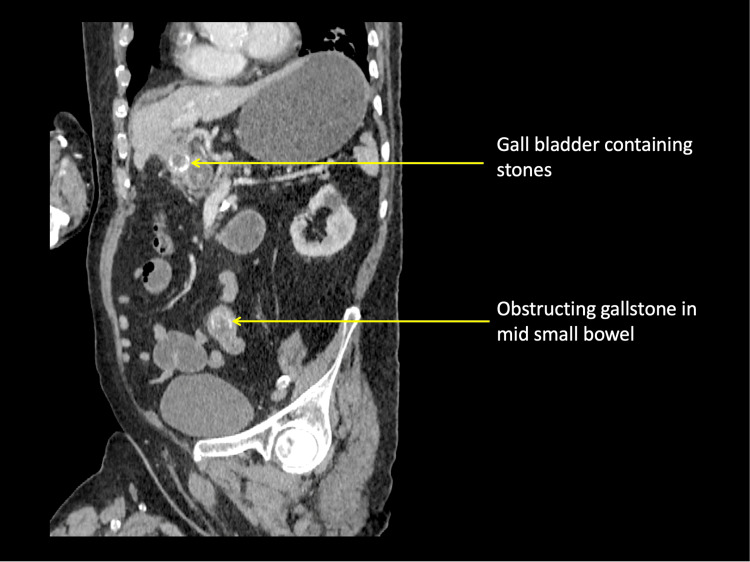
Coronal CT showing stones in the gall bladder. An obstructing gall stone in mid small bowel is noted.
